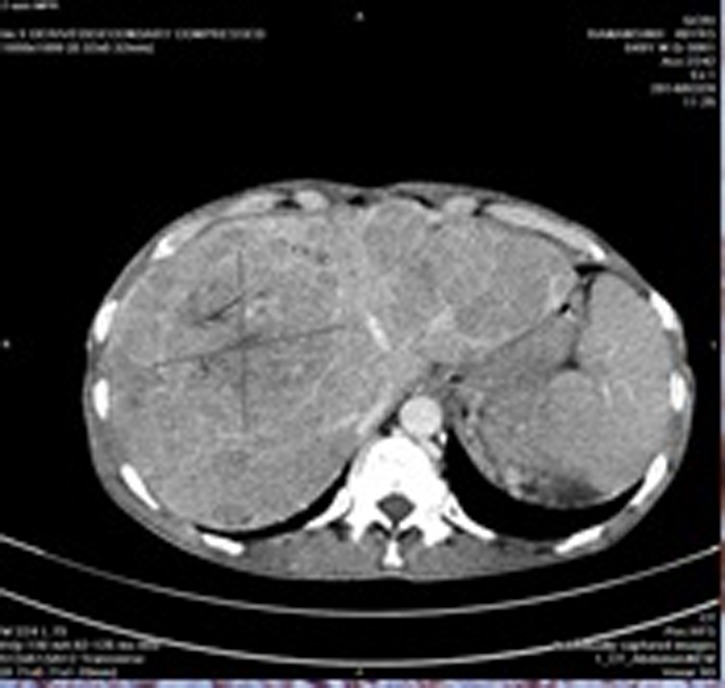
Abdomen CT showing heterogeneous echo texture lesions in right lobe of liver, measuring 83.8 x 84.6 mm in size.
